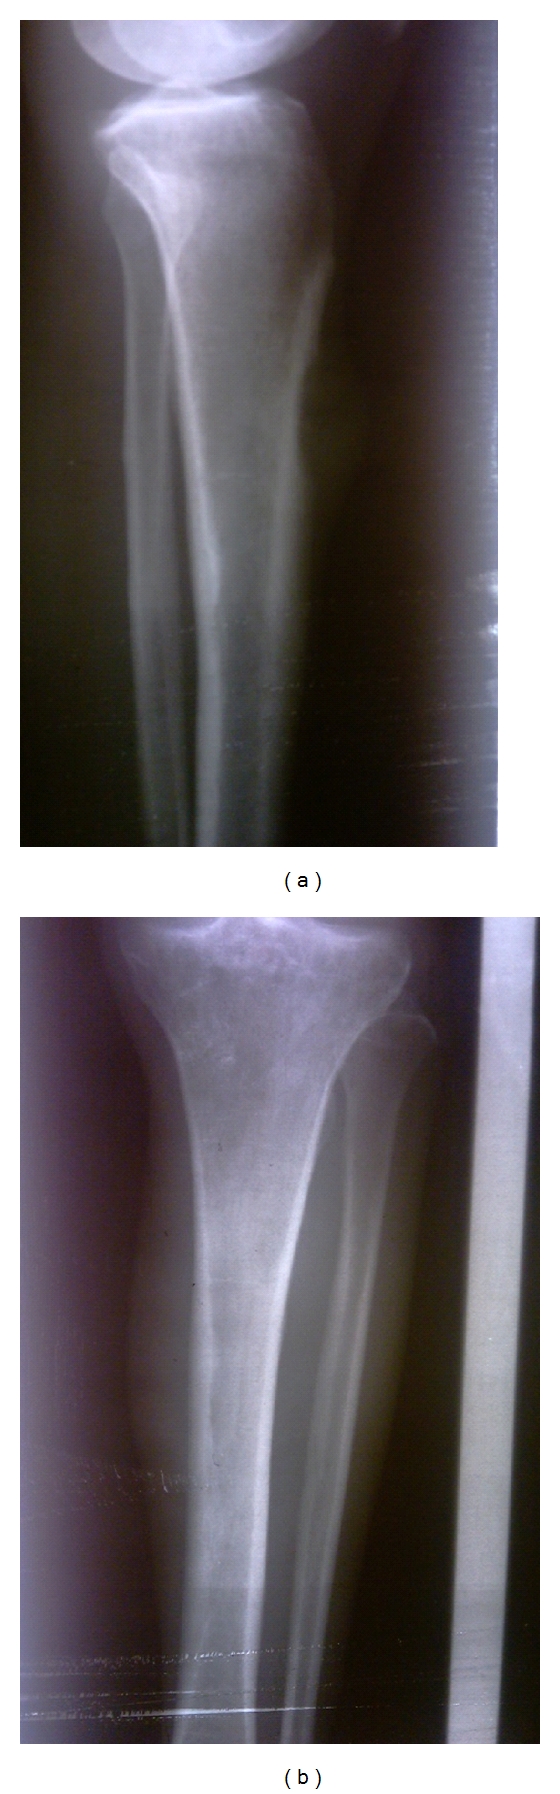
Simple X-ray of lower extremity. Slight thinning of the anterior cortex without cortical destruction.
